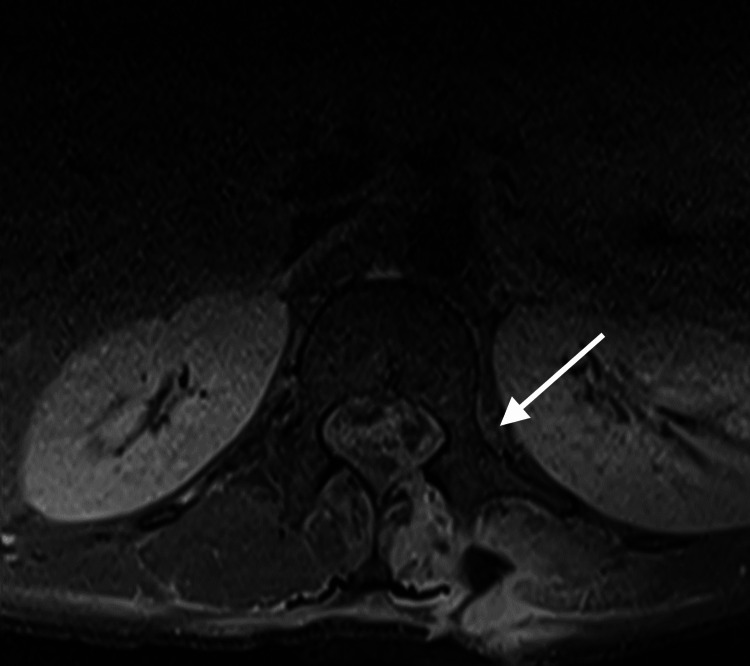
MRI T1 axial view of the stab at the L1 level
